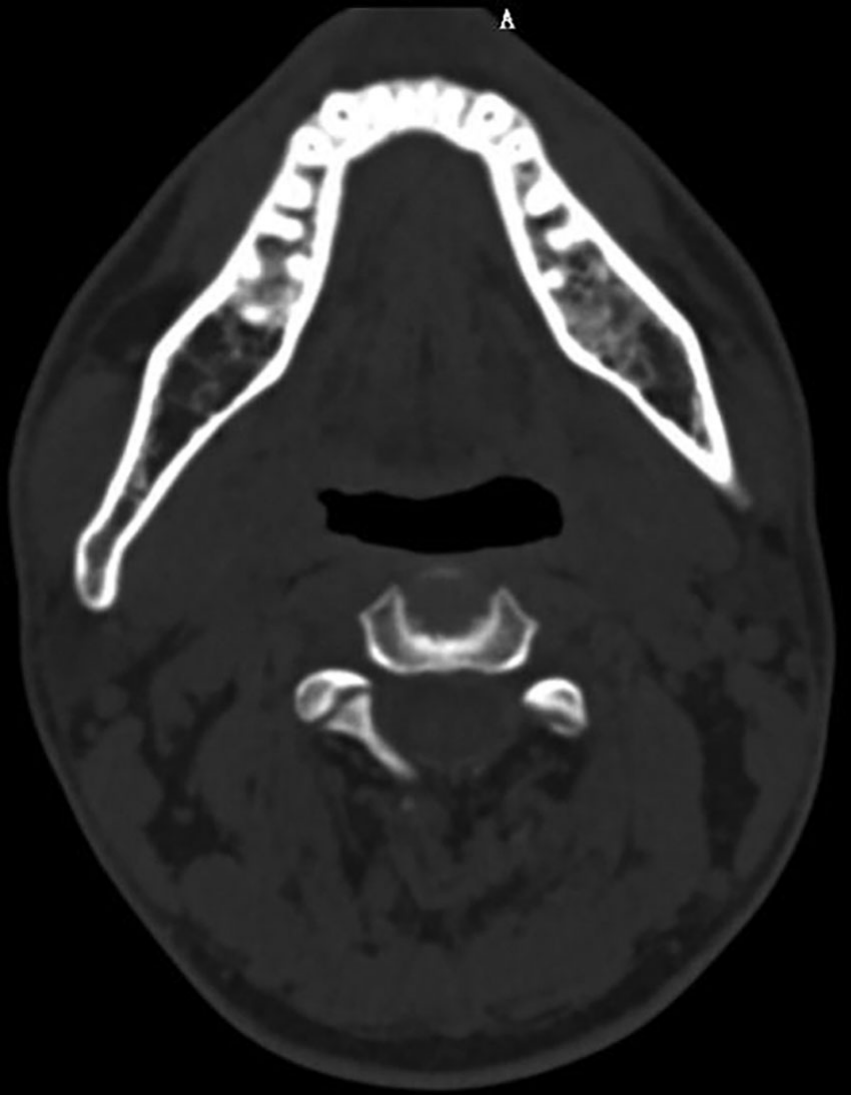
One example of face asymmetry by CT scanning.
